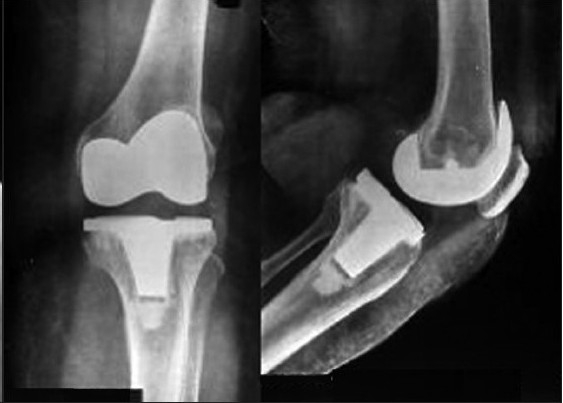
Anteroposterior and lateral radiographs at 18 month followup post mini-subvastus TKR of the same patient
